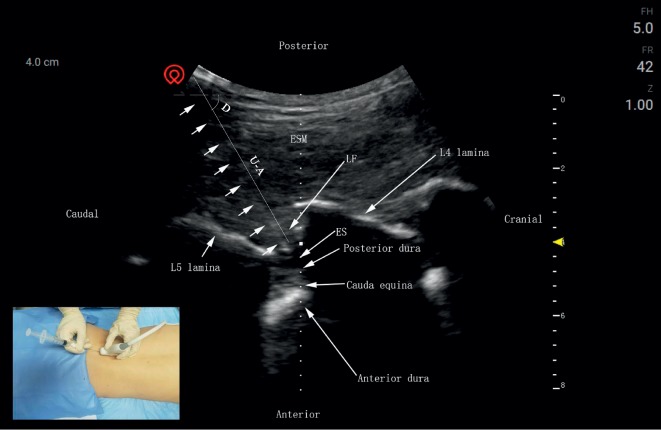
Longitudinal-axis approach: puncture needle of the paramedian sagittal sonogram of the lumbar spine. The posterior epidural space is a hypoechoic area between the hyperechoic ligamentum flavum and the posterior dura. The short arrows refer to the needle knife position. ESM, erector spinae muscle; ES, epidural space; LF, ligamentum flavum; U-A, the depth of acupotome penetration; D, the angle of acupotomy.
